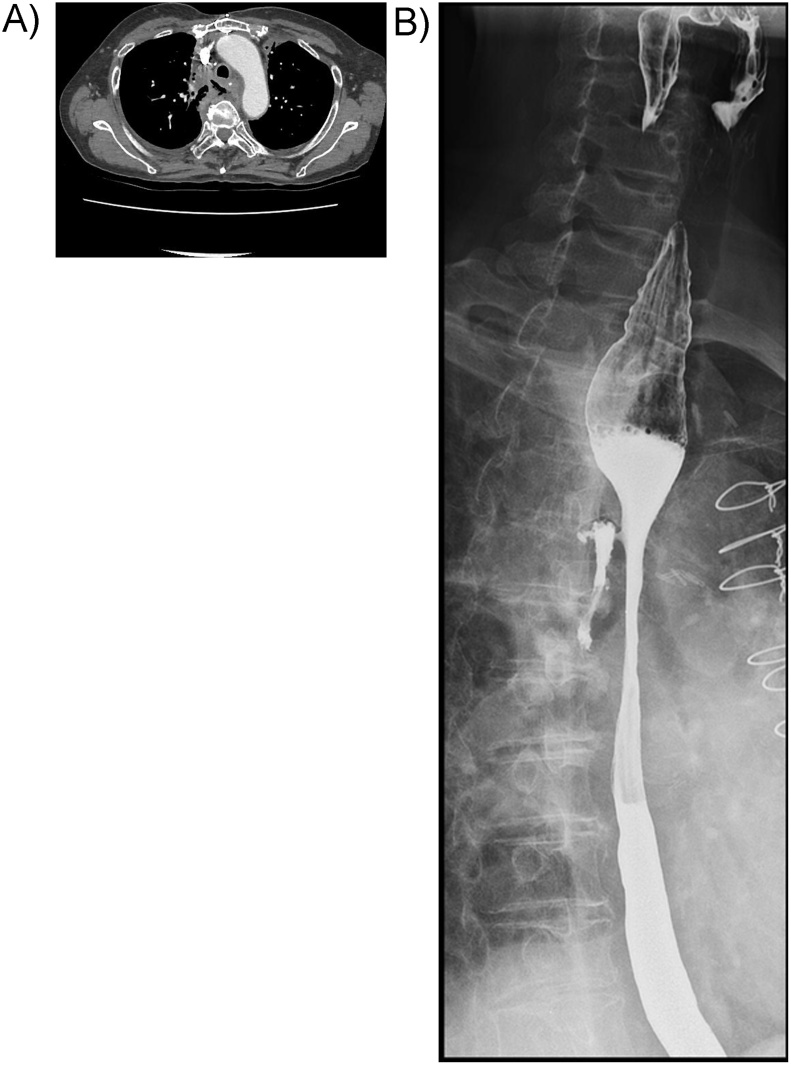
A: Enhanced chest computed tomography showing soft tissue infiltration with air-fluid collection around upper esophagus. B: Gastrografin esophagography showing esophagomediastinal fistula in the upper esophagus.
